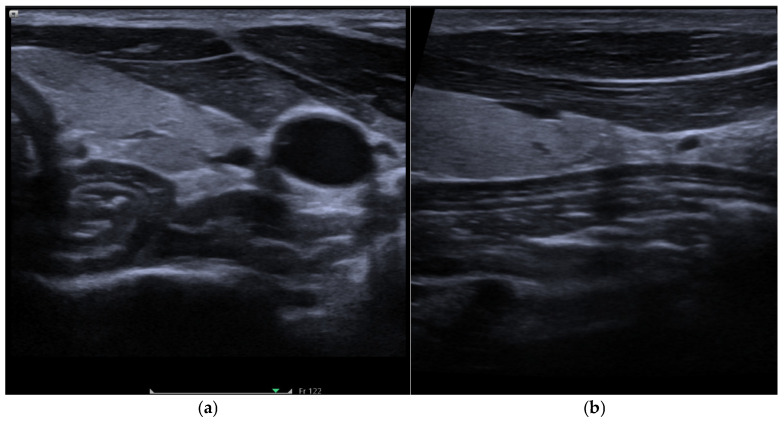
Representative B-mode ultrasound images of the cervical esophagus in (a) transverse view and (b) longitudinal view.
